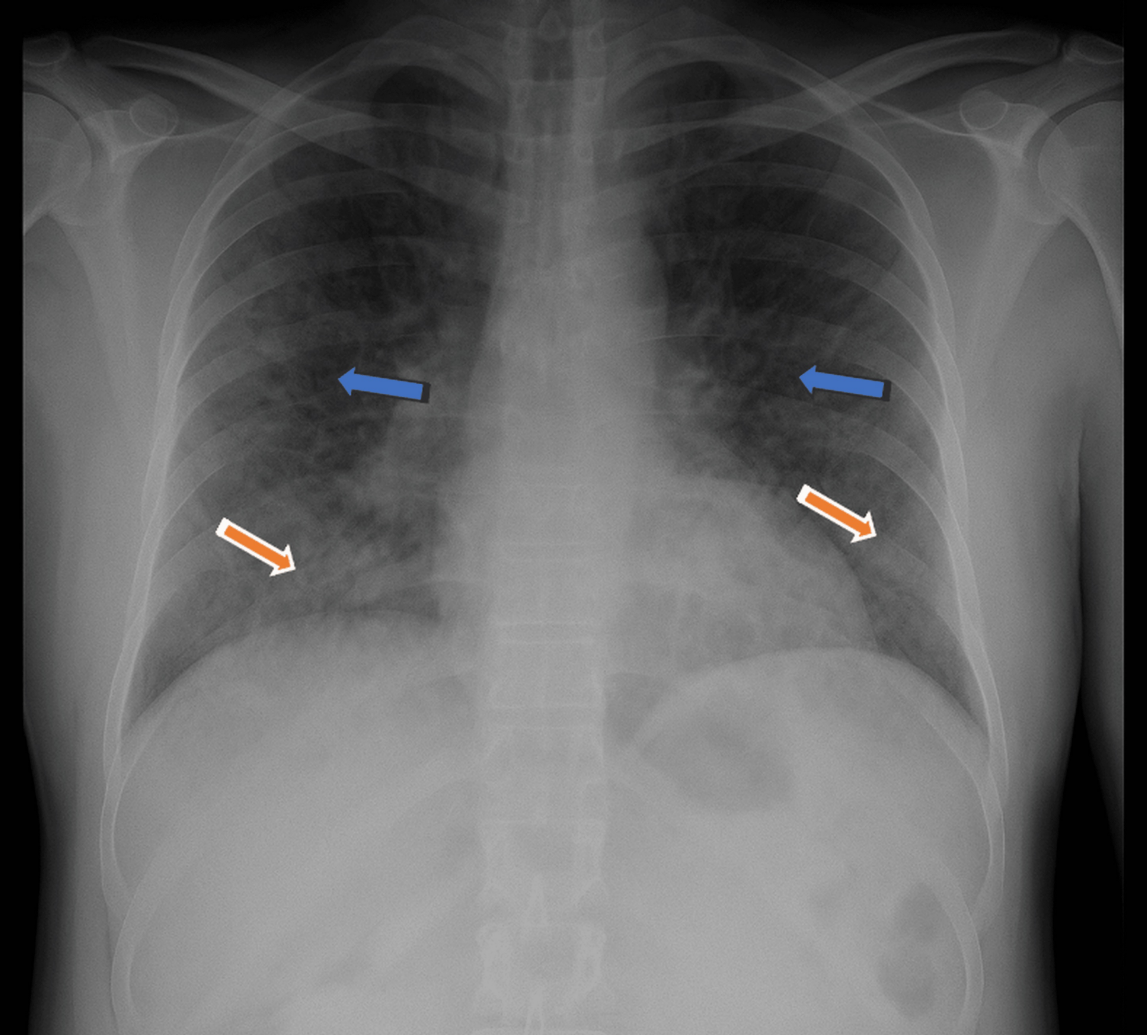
Chest X-ray showing diffuse bilateral interstitial (blue arrows) and airspace opacities (red arrows), more prominent in the lower lobes
